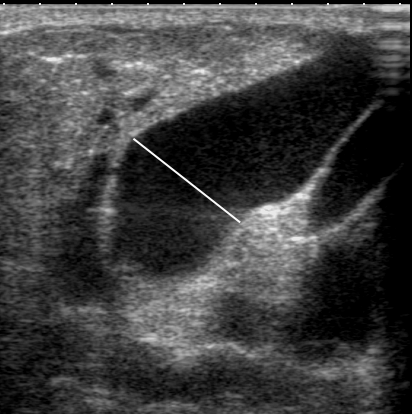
Ultrasound image of the main milk duct in a lactating woman. The main milk duct is displayed on ultrasound as a hypoechoic (black) structure with echogenic walls (white). This main milk duct is very large, measuring 9.2 mm in diameter.
